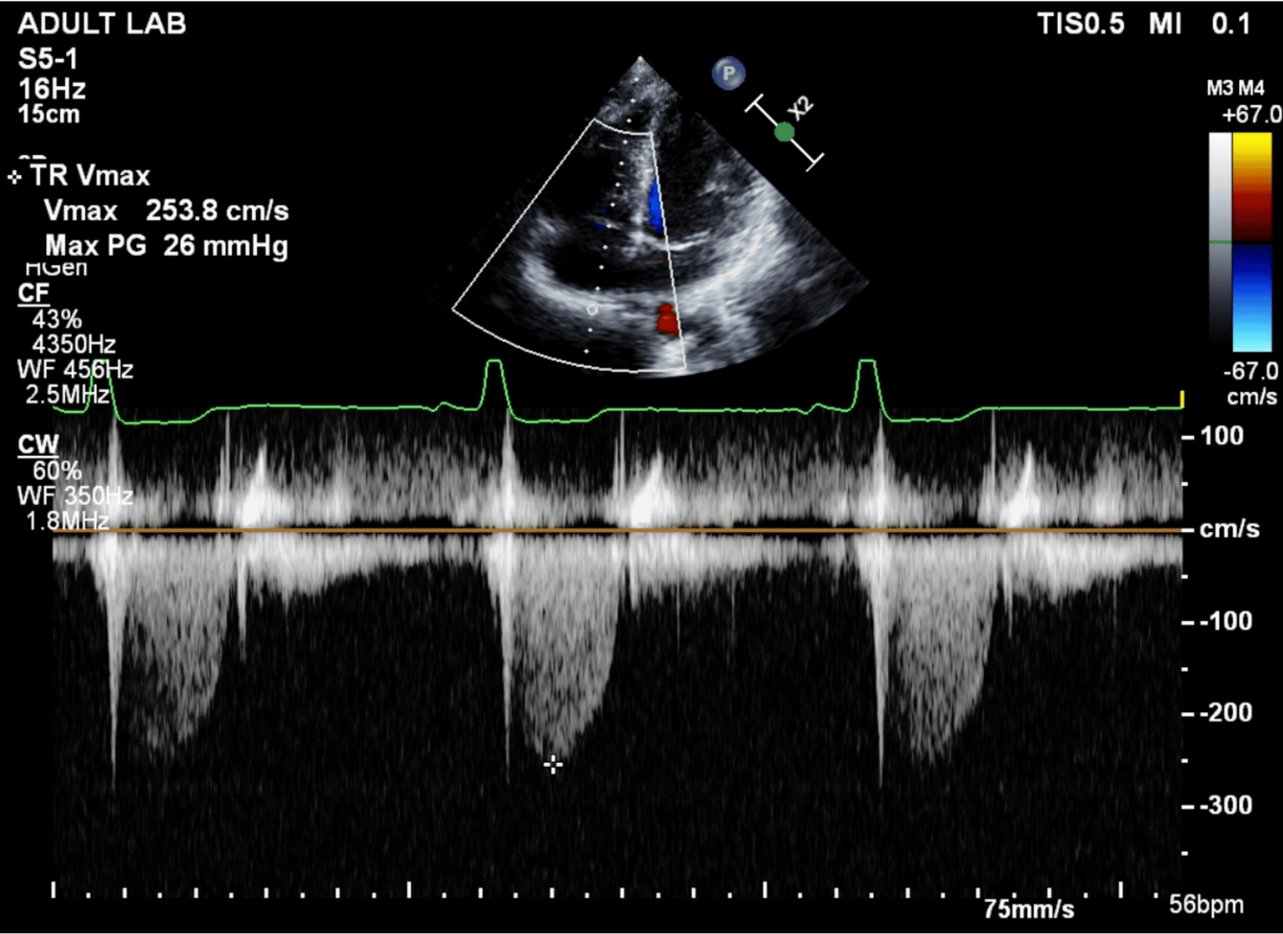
Continuous-wave Doppler echocardiography of tricuspid regurgitation suggesting a more than mild degree of regurgitation
